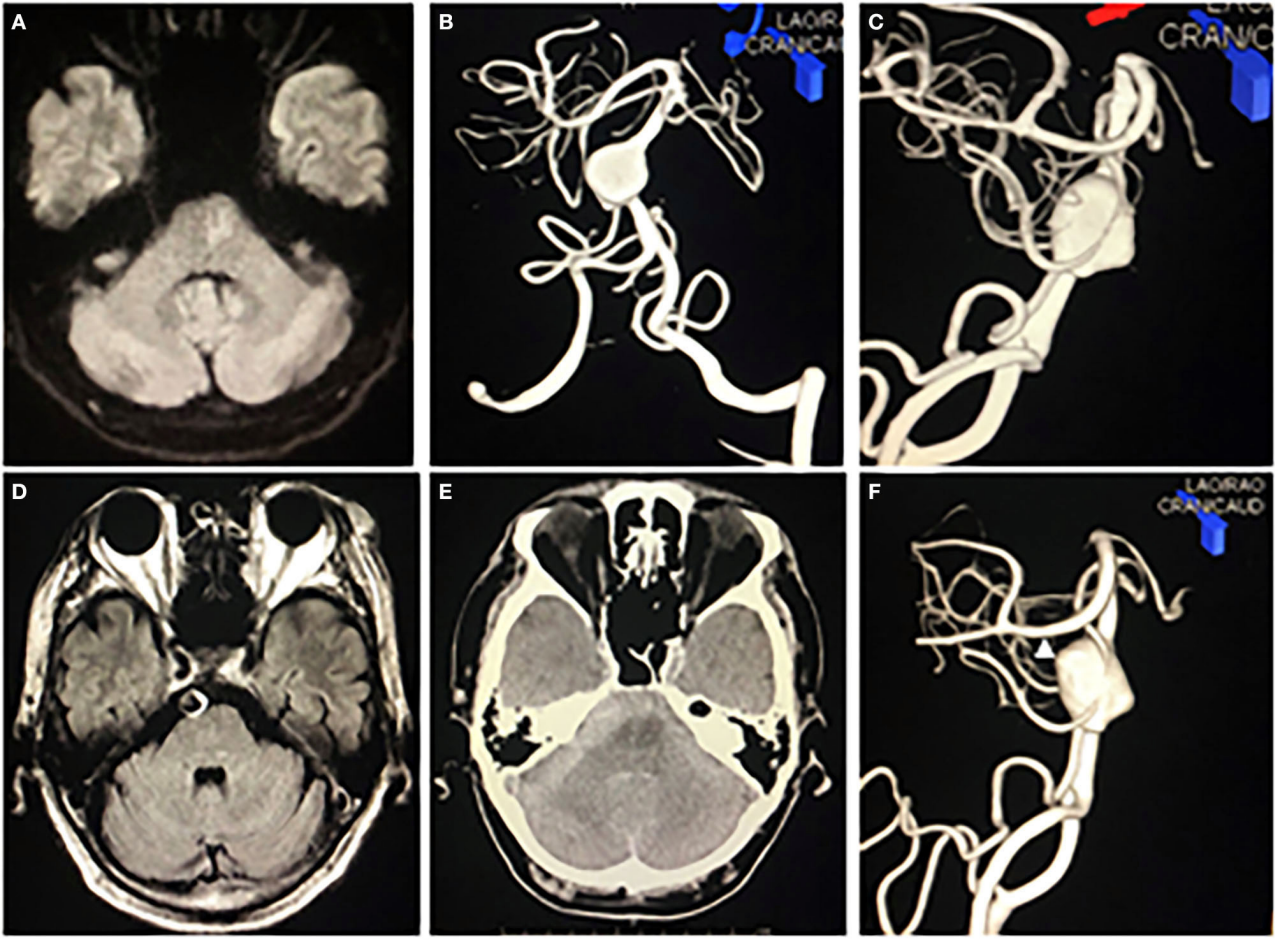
Preoperative imaging studies. (A) Diffusion-weighted image on MRI showed a small high-intensity lesion on left paramedian pons. (B,C) Anterior-posterior view (B) and right lateral view (C) of left vertebral artery multiplanar 3D rotational angiography revealed a giant fusiform basilar artery aneurysm. (D) A fluid-attenuated inversion recovery image on MRI revealed that the aneurysm was partially thrombosed. (E) CT showed diffuse subarachnoid hemorrhage incredibly thick in the right prepontine cistern. (F) Right lateral view of left vertebral artery multiplanar 3D rotational angiography revealed a bleb (white arrowhead) on the right shoulder of the fusiform basilar artery aneurysm.
